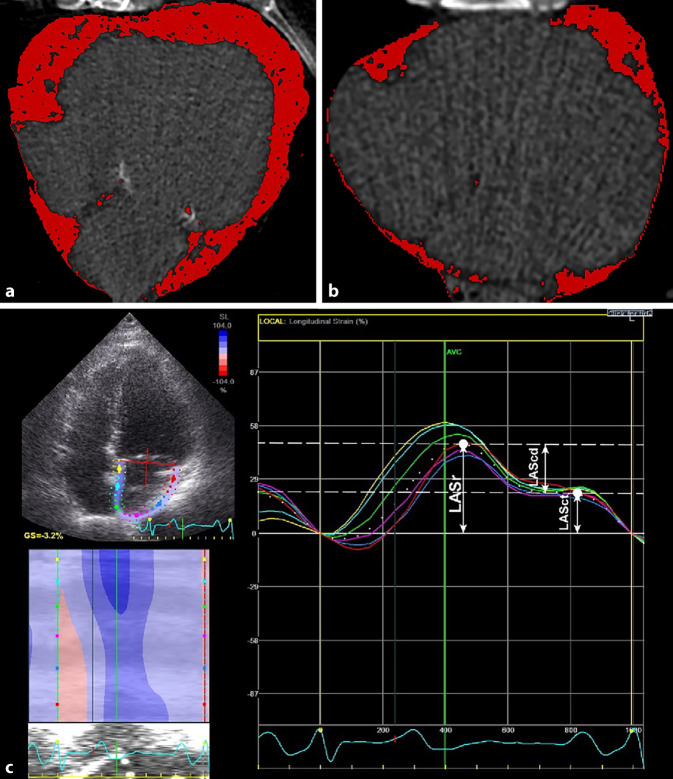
Assessment of epicardial adipose tissue volume by computer tomography (a, b). Patient A has a total volume of 476 ml and patient B 73 ml epicardial adipose tissue (*red*). Assessment of functional impairment of atrial deformation properties by speckle-tracking echocardiography (*left upper panel*) with focus on left atrial reservoir (*LASr*), conduit (*LAScd*) and contractile (*LASct*) strain (*left lower and the right panel*) (c)
